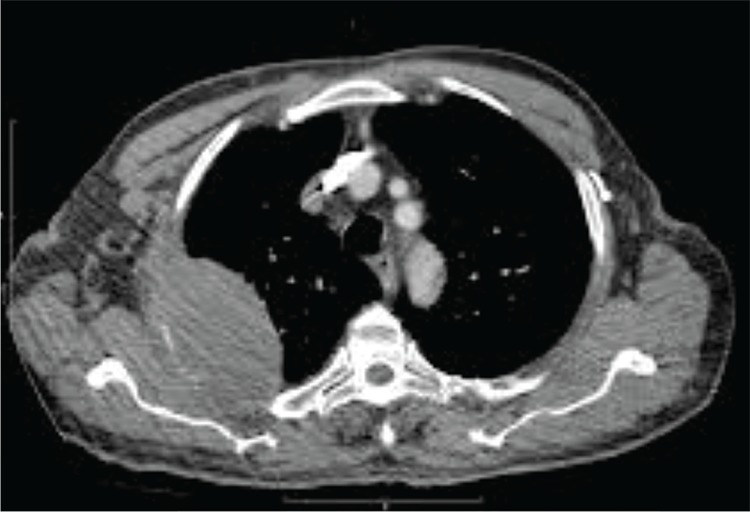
A 67-year-old male patient suffering from chest pain, cough, shortness of breath and extremity pain was referred to the department of radiology for imaging. Computed tomography of the chest showed a large mass in the right lung and biopsy cytology results were positive for adenocarcinoma. The patient was then referred to the department of nuclear medicine for whole-body bone scintigraphy (WBBS) due to extremity pain.
